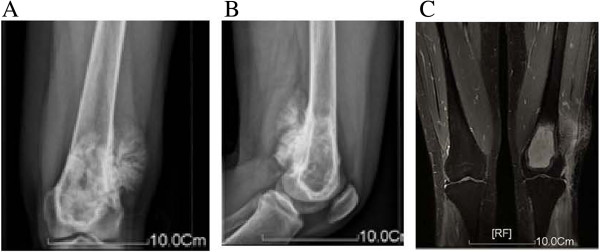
Data of radiography. A, B) Anteroposterior and lateral radiographs and C) magnetic resonance imaging studies were consistent with an osteosarcoma of the left distal femur.
